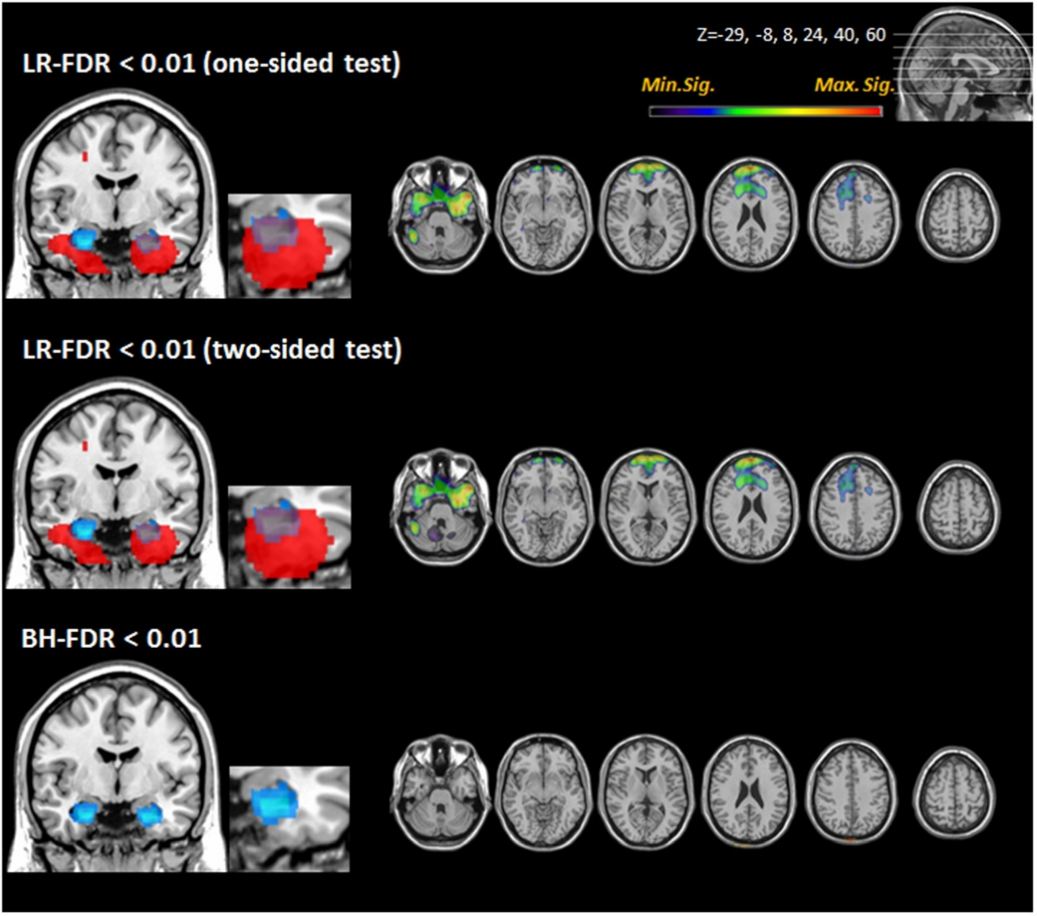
Brain regions with significantly lower FDG uptake in QD compared to NC. The coronal view in the left column shows hypometabolism in the medial temporal regions in QD. An anatomical map of the hippocampus is displayed in blue. Regions with a lower FDG uptake are displayed in red. The LR-FDR one-sided and two-sided tests disclosed more extensive hypometabolic areas in both temporal lobes than did the BH-FDR method. (FDR: False discovery rate; LR-FDR: Likelihood ratio false discovery rate; NC: Normal controls; QD: Questionable dementia).
